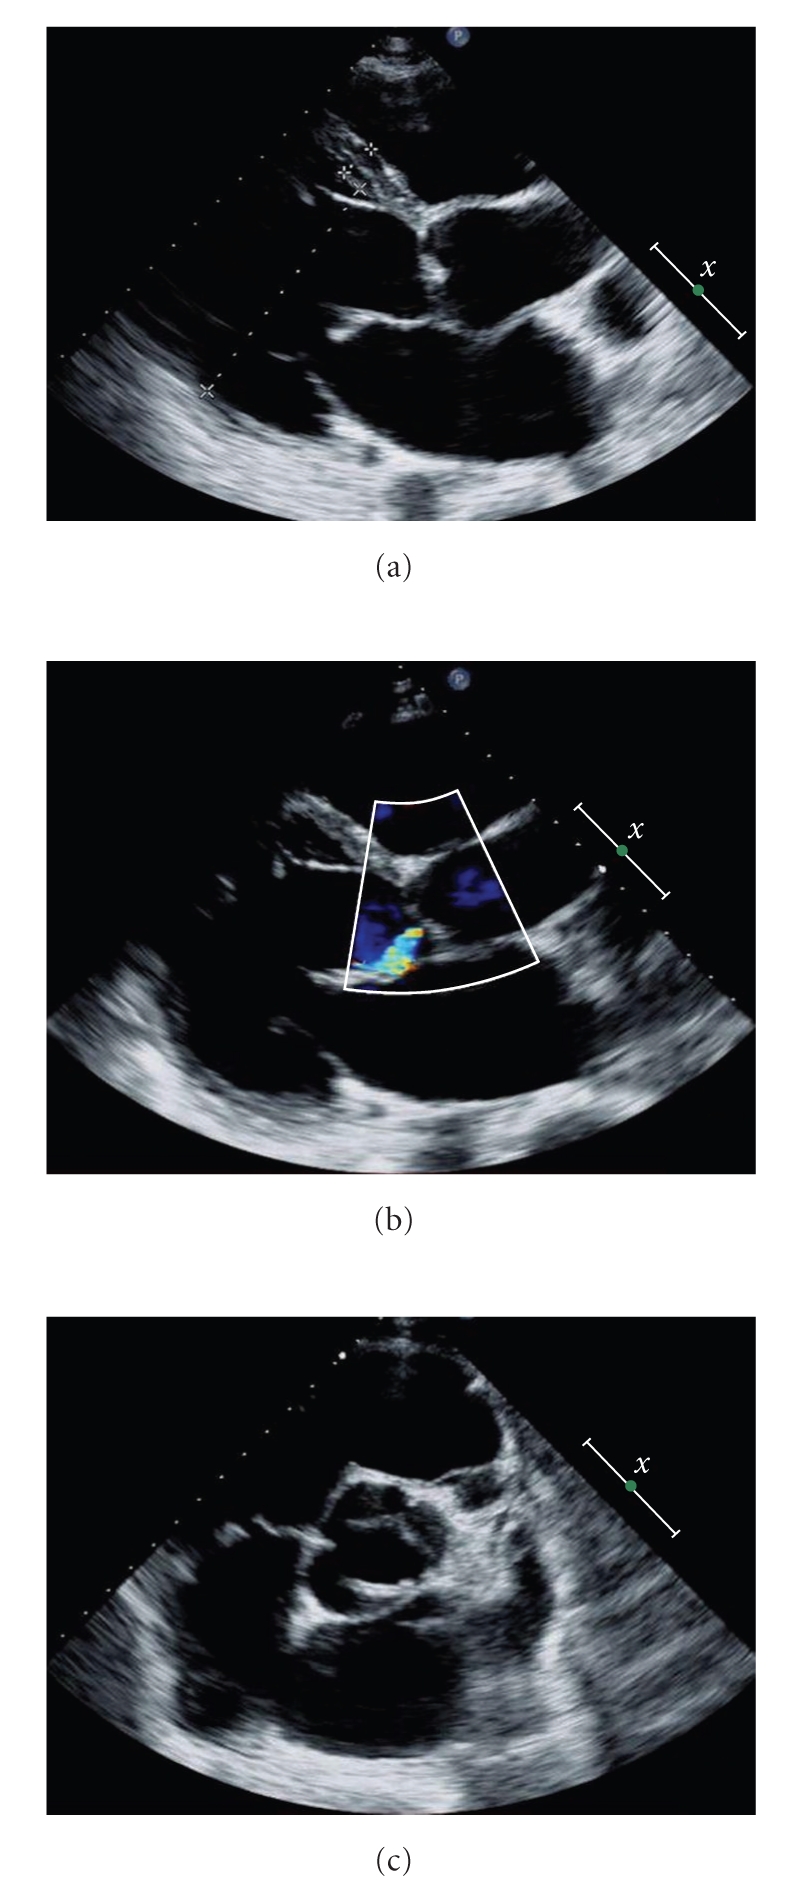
Transthoracic echocardiography. (a) Parasternal long-axis view, the left ventricule telediastolic diameter is 80 mm. (b) Parasternal long-axis view, aortic regurgitation. (c) Short-axis view at the level of the aorta, bicuspid aorti valve.
